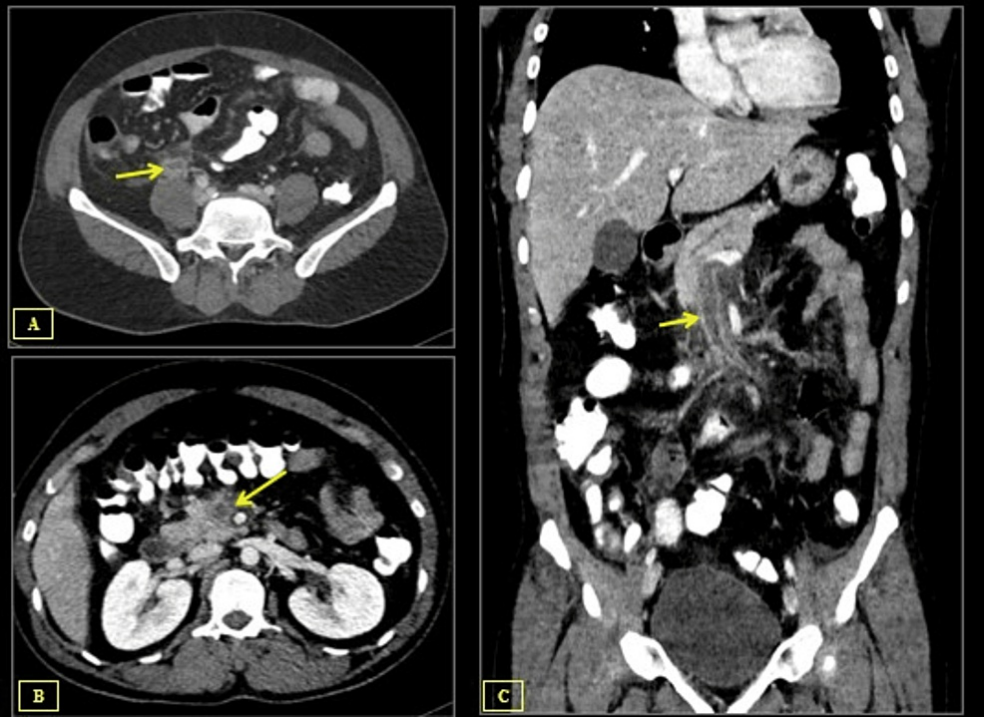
Multiple sections of axial and coronal contrast-enhanced CT of the abdomen in the portal venous phase (A) Axial contrast-enhanced CT abdomen shows an enlarged and thick-walled appendix (arrow), along with evidence of surrounding fat stranding. (B) Axial contrast-enhanced CT abdomen reveals a low attenuation thrombus within the superior mesenteric vein lumen (arrows). (C) Coronal contrast-enhanced CT abdomen reveals a low attenuation thrombus within the superior mesenteric vein lumen (arrows).
